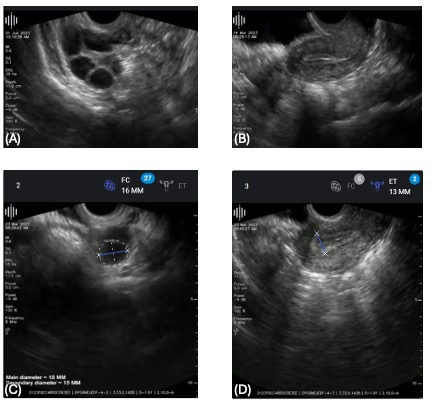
Demonstration of typical scans of (A) follicles and (B) the endometrium performed by the self-operated FC transvaginal device. Measurement tools are available in the clinician dashboard and are used for measurement of (C) follicle size and (D) endometrial thickness. FC: Pulsenmore follicle count home vaginal ultrasound.
